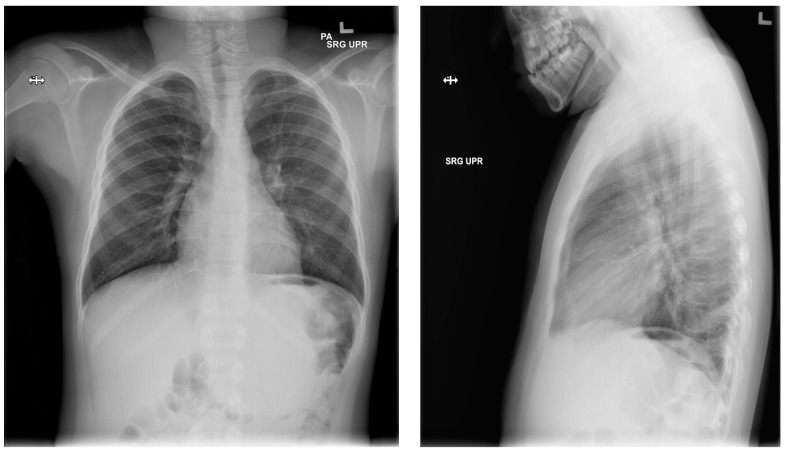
Most recent chest X-ray, several months after removal of shunt.
